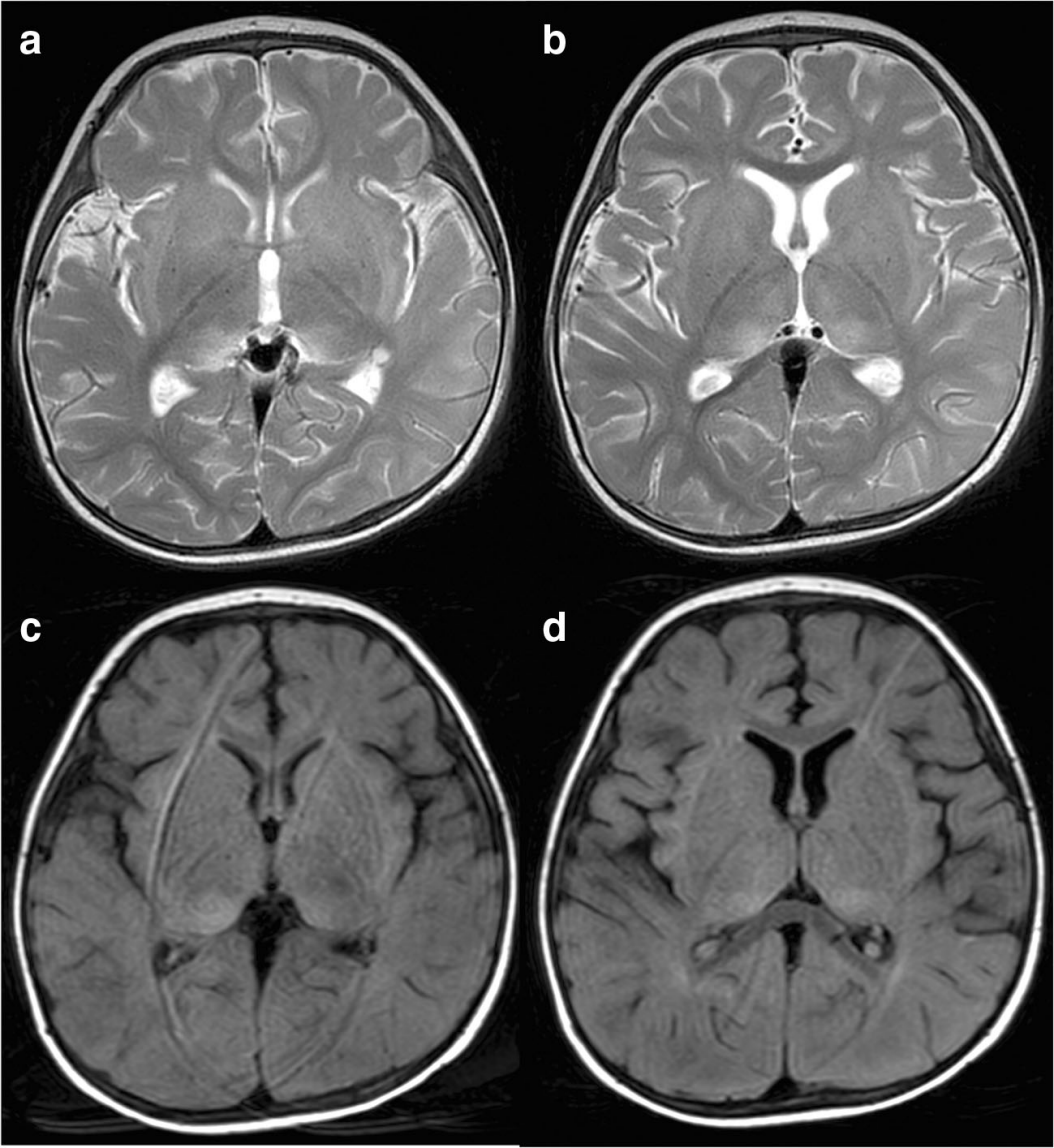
Brain MRI obtained at day 4. a, b The axial T2 weighted image (T2WI) showed high intensity regions in the bilateral thalamus. c, d The axial fluid attenuation inversion recovery (FLAIR) image showed high intensity regions in the bilateral thalamus
